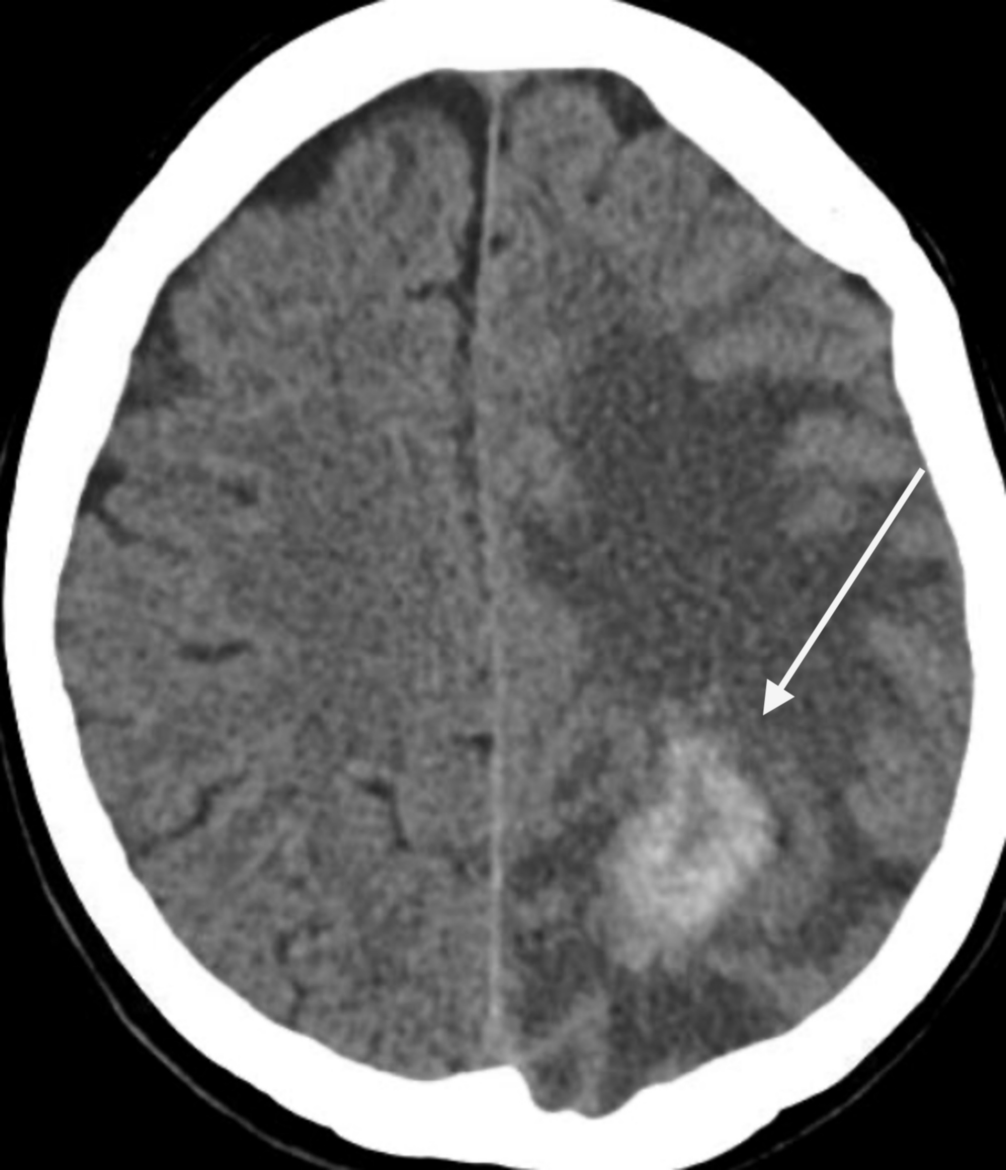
CT of the head without contrast. CT of the head without contrast showed a right-sided frontal and left-sided parietal hemorrhagic mass with a large amount of vasogenic edema associated with moderate mass effect from left to right.
